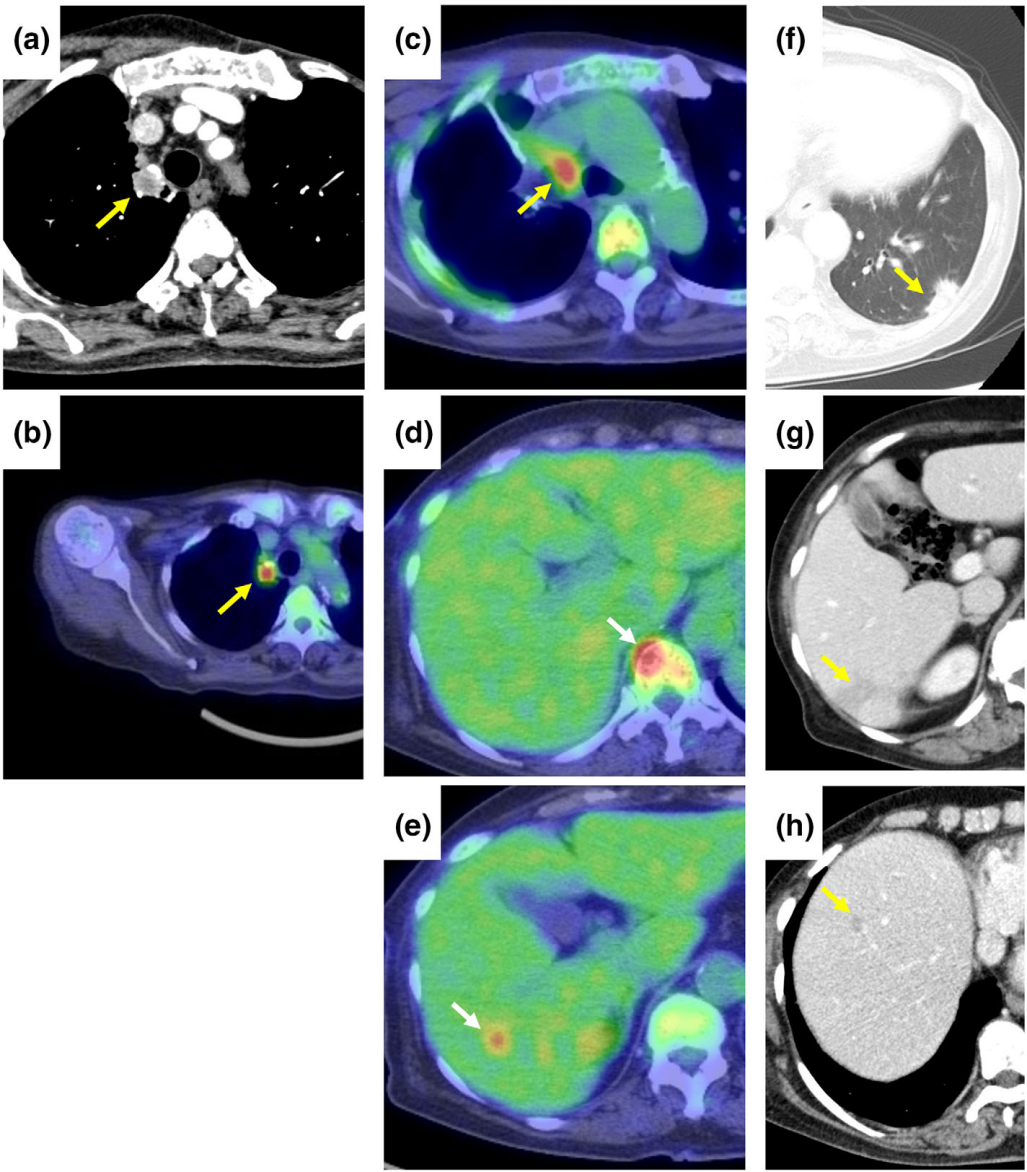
(a) and (b) Before right upper lobectomy. (a) Chest computed tomography (CT) with a 15.6‐mm nodule (arrow) localized in the right upper lobe. (b) Fluorodeoxyglucose‐positron emission tomography (FDG‐PET) CT with a nodule (arrow) localized in the right upper lobe without distant metastasis. (c)–(e) Postoperative FDG‐PET CT. FDG uptake is seen in mediastinal lymph nodes (c), bone (d), and liver (e). (f)–(h) CT after erlotinib and ramucirumab therapy. A new pulmonary lesion (f) and some liver lesions (g) and (h) are seen
